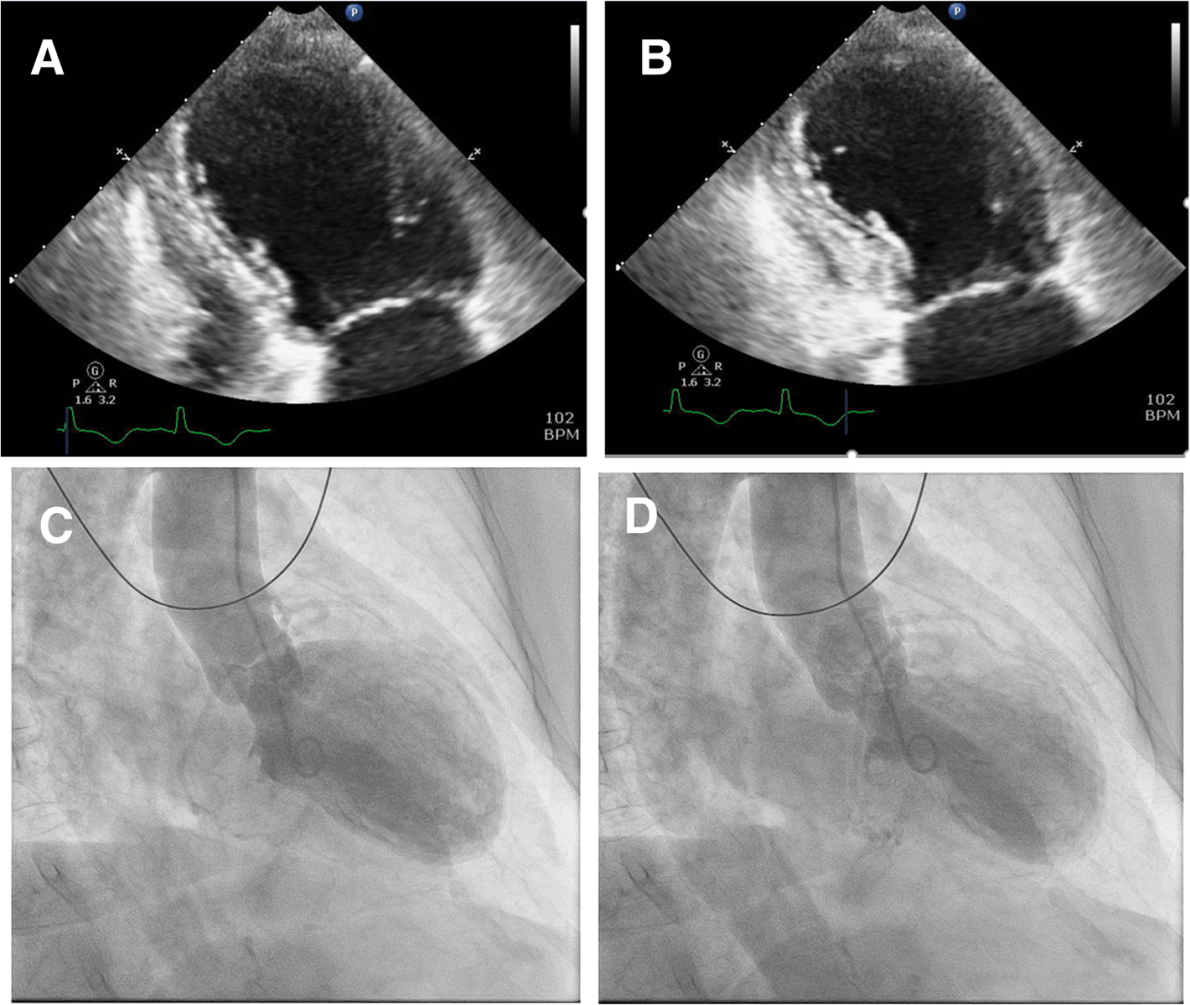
Echocardiogram pattern in a patient with TCM demonstrating apical ballooning and diffuse hypokinesis with sparing of the basal myocardial segments. Left ventricle in (a) end-diastole and (b) end-systole is shown; Left Ventriculogram pattern in TCM shown in (c) end-diastole and (d) end-systole
